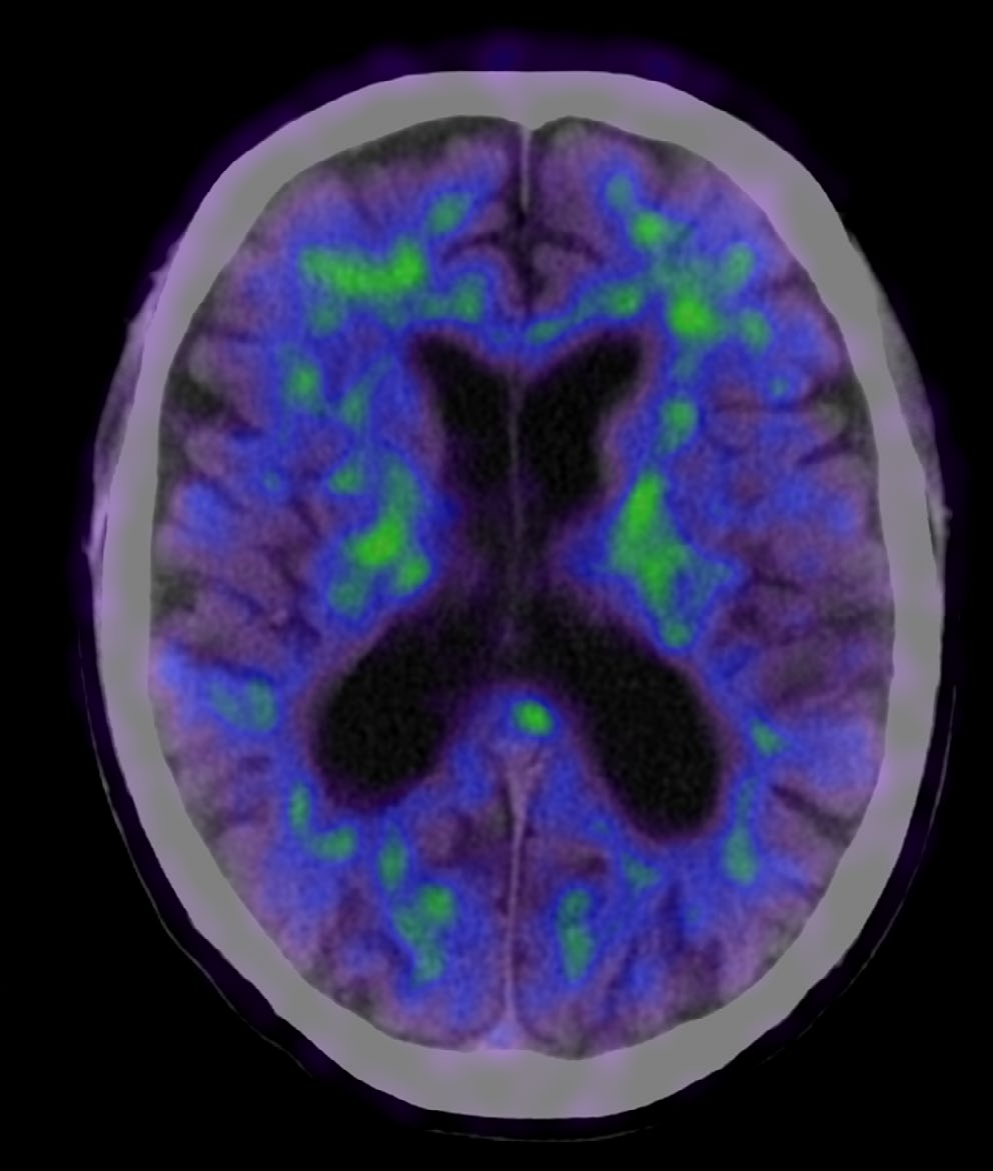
Brain [^11^C]PiB‐PET. The tracer has high affinity for β‐amyloid plaques. The PET scan is overlaid on CT and shows unspecific tracer retention in white matter, but no tracer binding in striate or cortex. Region of interest analysis also showed a normal cerebral to cerebellar cortical standardized uptake value (SUV) ration of 1.02 (normal < 1.5)
